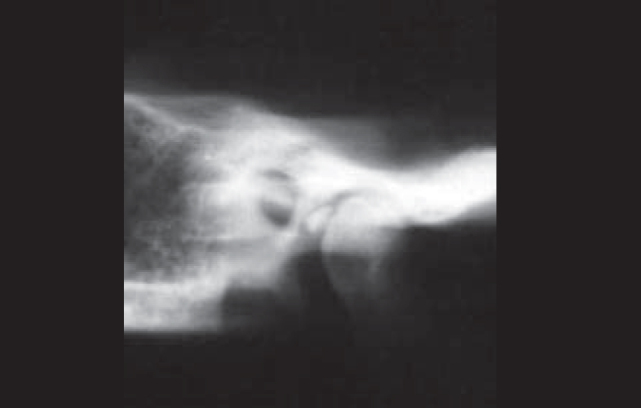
Corrected lateral CTs can - TMJ CT scan sections - section C with gutta-percha.
